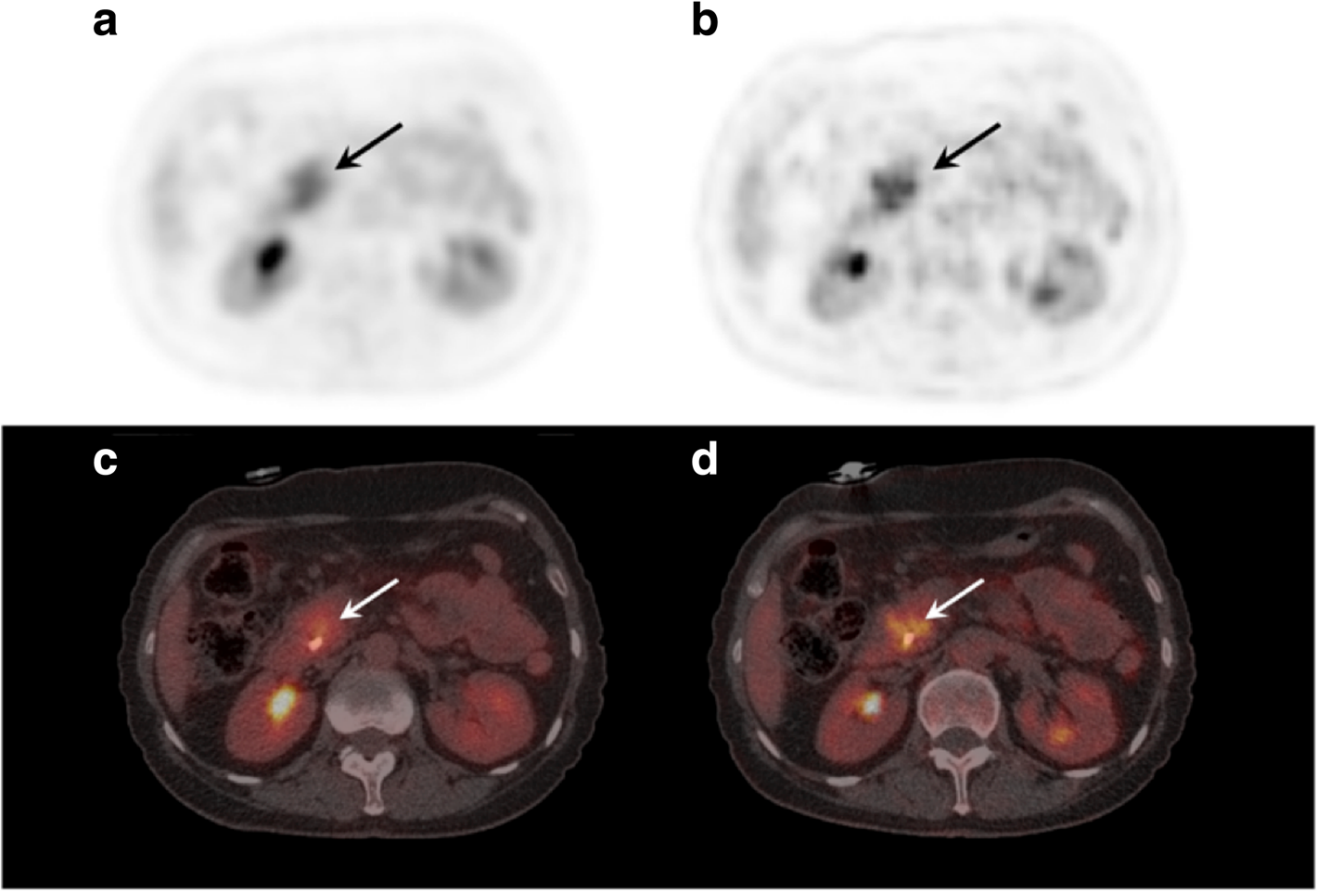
Optimal respiratory gating impacts assessment of intra-tumoral heterogeneity. A [^18^F]FDG PET/CT scan reconstructed according to EANM guidelines (a, c) and with optimal respiratory gating (b, d) showing changes in the heterogeneous pattern of intra-tumor [18F]FDG uptake
